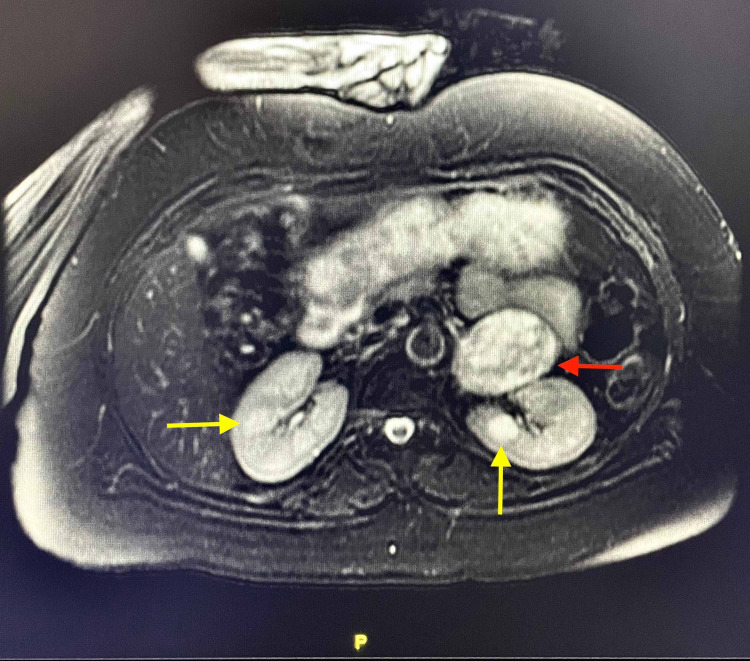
Axial view- T2-weighted MRI with fat suppression showing a left adrenal, well-circumscribed mass, measuring 4.4 x 5.7 x 5.1 cm (red arrow), and bilateral renal cysts (yellow arrows) MRI: magnetic resonance imaging
